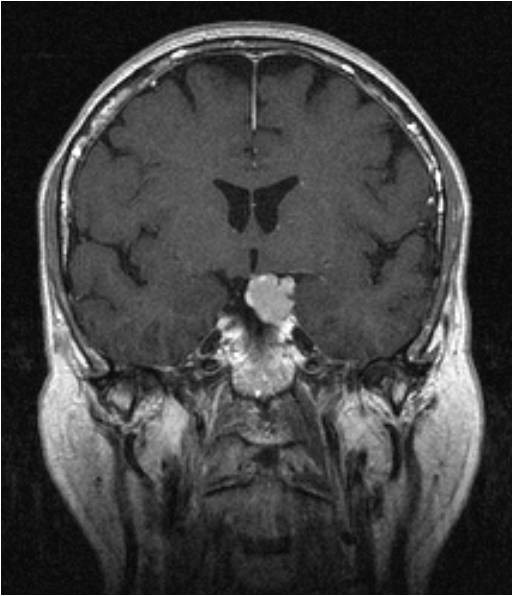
MRI, T1 weighted images after administration of gadolinium, coronal section, demonstrates the circumscribed skull base tumor.
